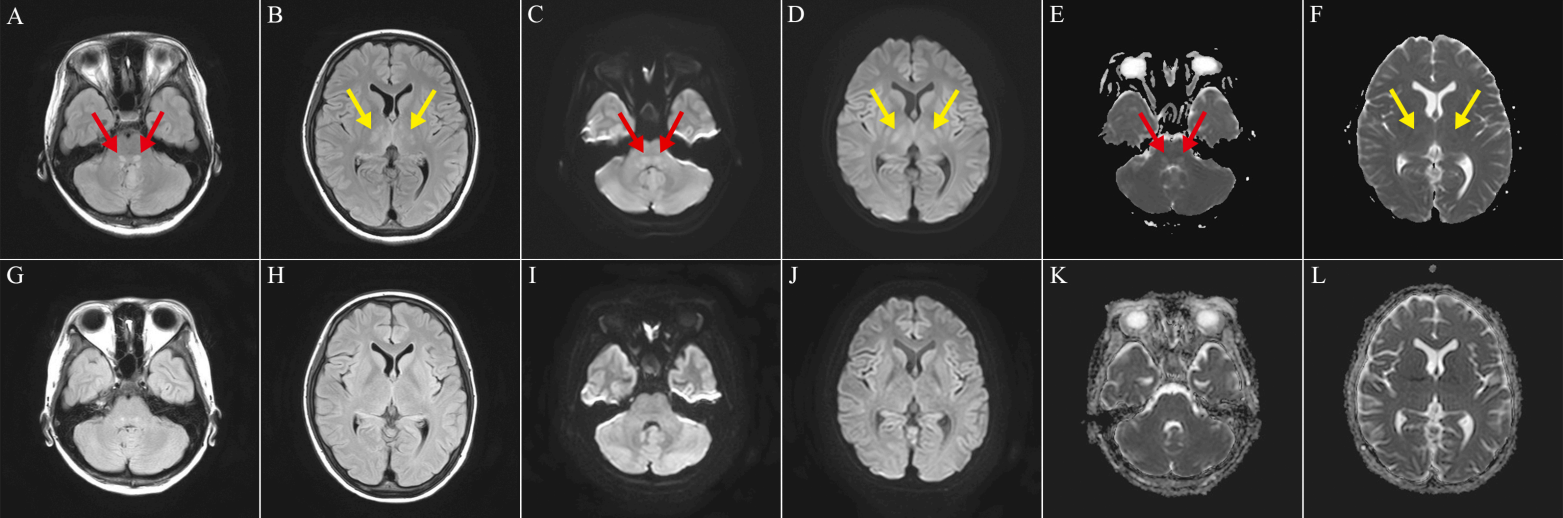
(A-F) Axial brain magnetic resonance imaging (MRI) of the patient showed bilateral symmetrical hyperintensities in the dorsal pontine base (red arrows) and thalamus (yellow arrows) on day 5 of hospitalization (A and B, T2-fluid-attenuated inversion recovery [FLAIR]; C and D, diffusion-weighted imaging; and E and F, apparent diffusion coefficient maps). (G-L) MRI signal changes in the pons and thalamus improved on day 12 of hospitalization (G and H, T2-FLAIR; I and J, diffusion-weighted imaging; and K and L, apparent diffusion coefficient maps).
